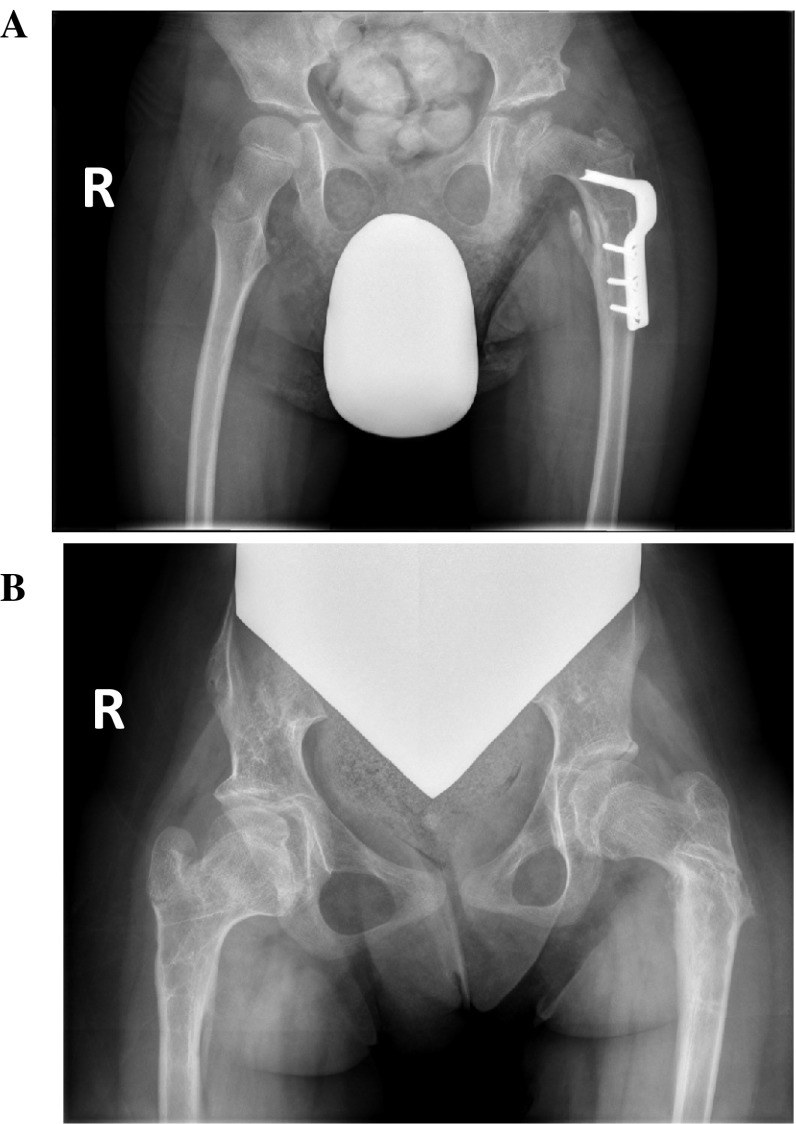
Optimal and suboptimal agreement between pediatric orthopaedic surgeons on the presence of avascular necrosis (AVN) of the femoral head in children with cerebral palsy. Anteroposterior radiographs of the hips were identified to have AVN by a 4/4 surgeons (*left hip*), and b 2/4 surgeons (*right hip*)
